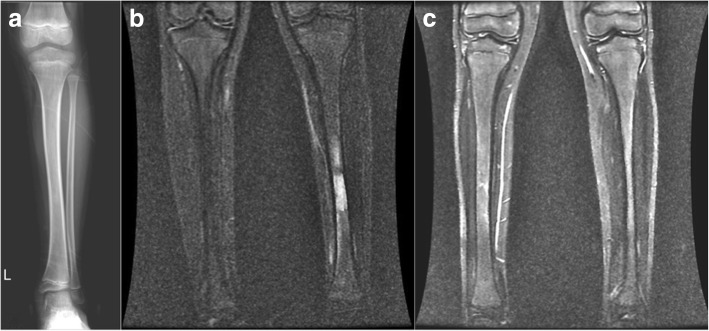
a Conventional radiograph of the 7-year-old patient diagnosed with transient edema in the tibia. b Coronal T2-weighted MR image. c T2-weighted MR image
